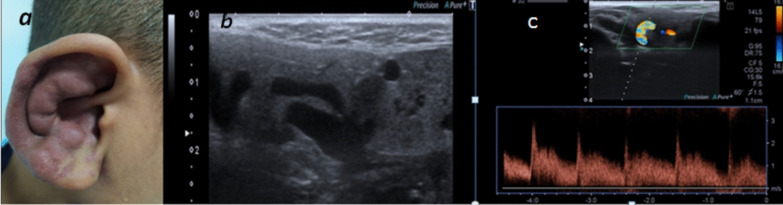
a 7-year-old male patient: Image of right auricle showing swelling and discoloration of the right auricle, b U/S image showing dilated serpigenous structures, c color Doppler of the lesion showing high flow vascular lesion with aliasing artifacts with PSV reaching 2.8
